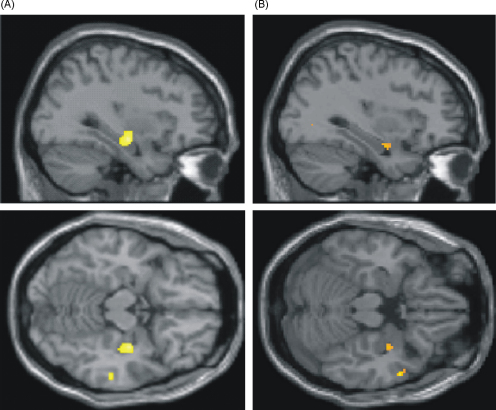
Anterior hippocampal grey matter volume differences between taxi drivers and control subjects. (A) Anterior hippocampal grey matter volume was decreased in licensed London taxi drivers compared with matched control subjects. Data are shown on sagittal (upper panel) and axial (lower panel) sections from the canonical SPM5 MRI scan. (B) Anterior hippocampal grey matter volume was negatively correlated with navigation experience, with less grey matter volume the more years spent taxi driving. Data are shown on sagittal (upper panel) and axial (lower panel) sections from the canonical SPM5 MRI scan.
